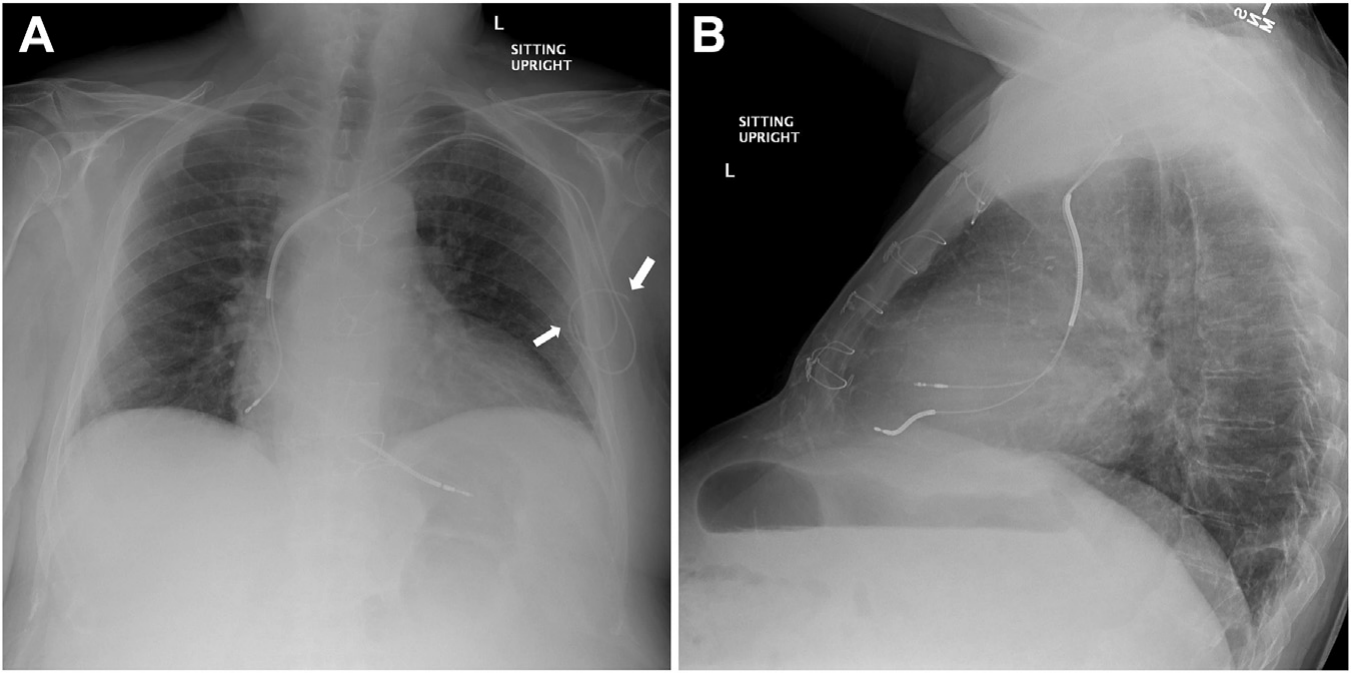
A: Posteroanterior chest radiograph. The severed leads are marked by white arrows. B: Lateral chest radiograph.
